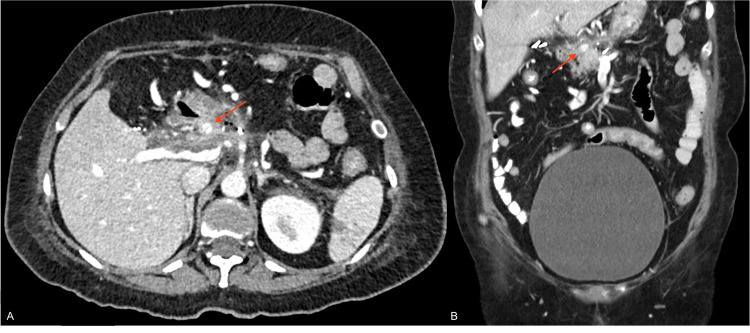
Contrast Enhanced CT scan images in axial (A) and Coronal (B) planes shows inflammatory changes in the surgical site of the partial pancreatectomy. Additionally, a redounded-like circumscribed enhancement is seen as compatible with a pseudoaneurysm (Arrows).
